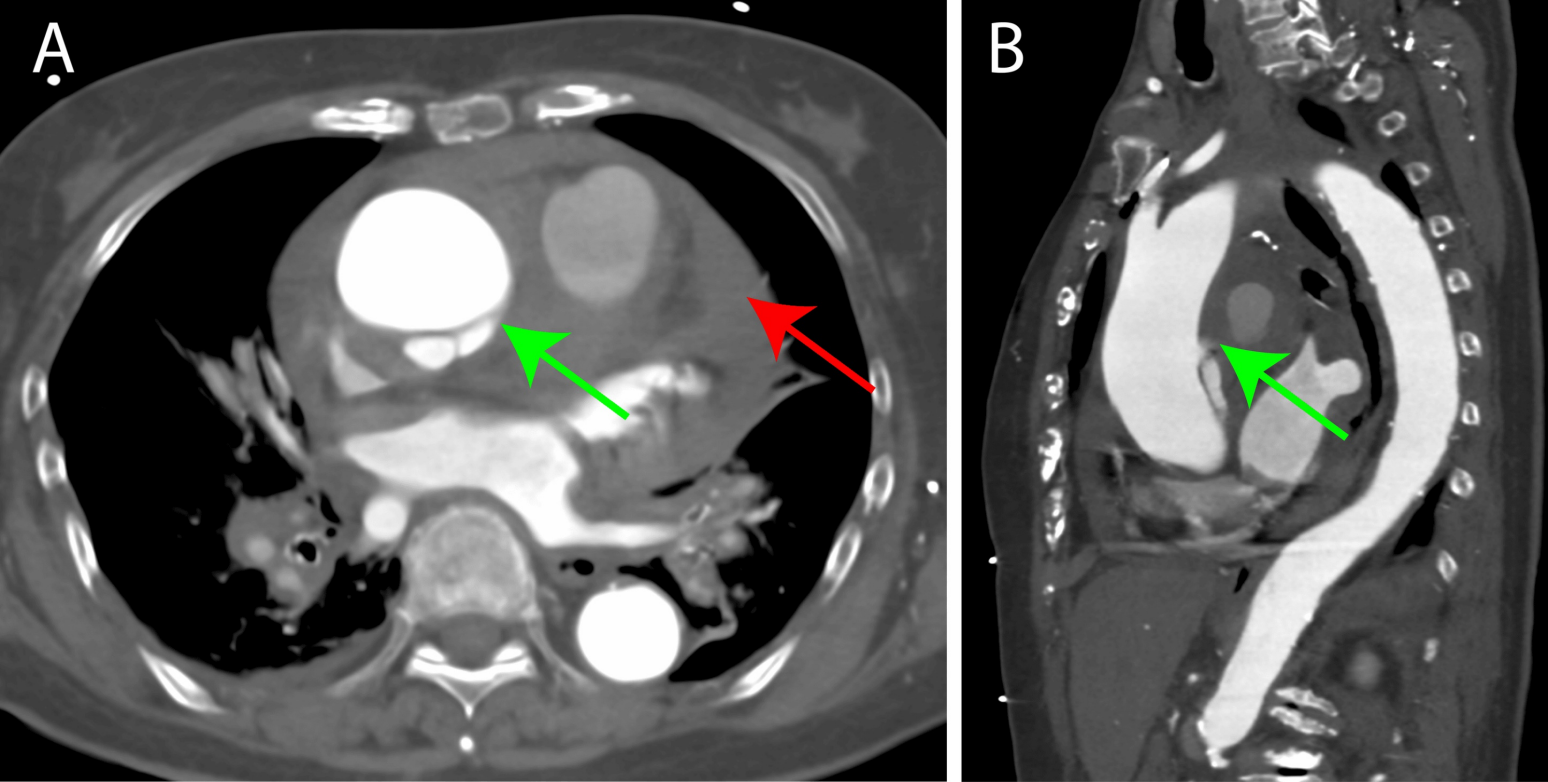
Preoperative computed tomography findings (A) The horizontal section displays an entry of dissection in the dorsal aspect of the ascending aorta (green arrow) and an intrapericardial hematoma (red arrow). (B) A sagittal section demonstrating dissection entry on the dorsal aspect of the ascending aorta (green arrow).
